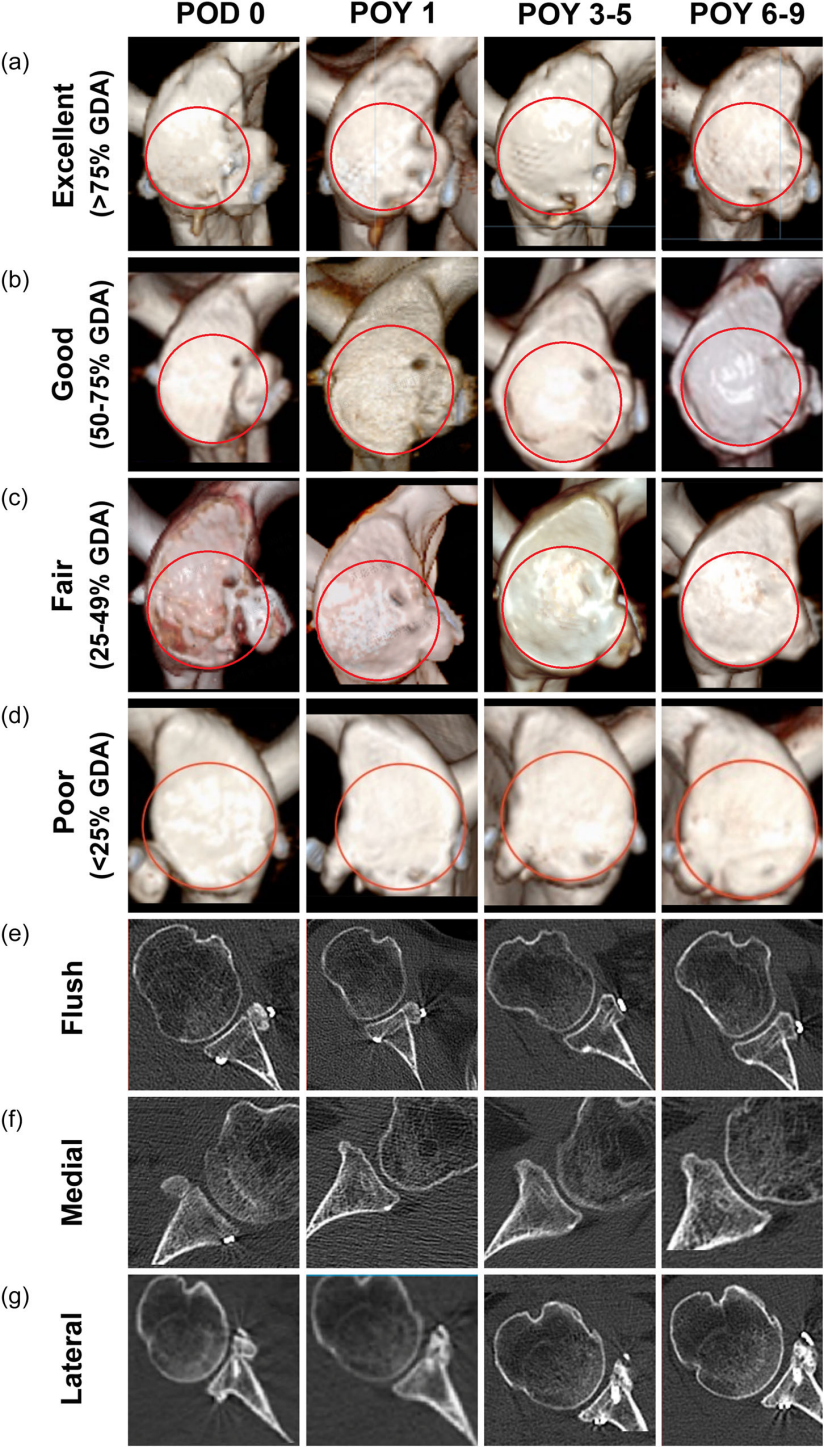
Remodelling of the CG‐glenoid interface of four representative excellent, good, fair and poor vertically positioned cases on the 3D CT en face view (a–d), and three representative flush, medial and lateral horizontal positioned cases on the 2D CT axial view (e–g) at different follow‐up time points. The red circle indicates the PFC of the glenoid. 2D, two‐dimensional; 3D, three‐dimensional; CG, coracoid graft; CT, computed tomography; GDA, glenoid defect area; PFC, perfect fitting circle; POD, postoperative day; POY, postoperative year.
